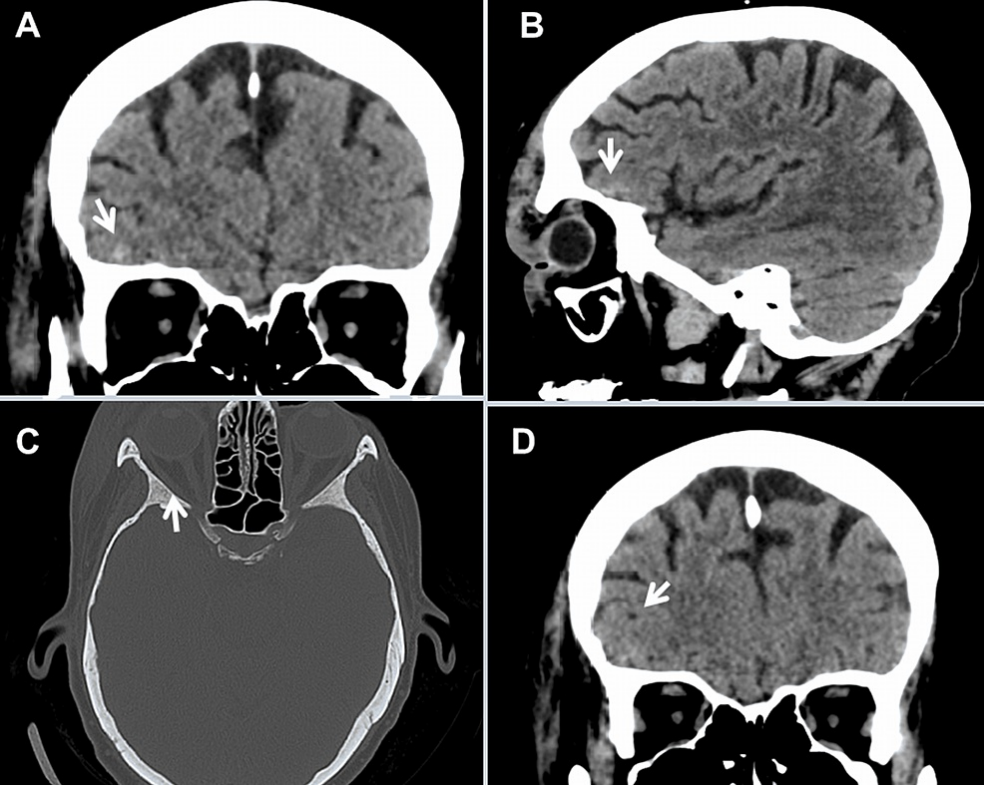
Cerebral CT scan showing hyperdensity in the right fronto-polar region (coronary view (A, D), sagittal view (B), and bone window (C)) being interpreted as subarachnoid bleeding. Since computed tomography angiography did not disclose an aneurysm and since the history was positive for a fall, the lesion was interpreted as traumatic subarachnoid bleeding.
